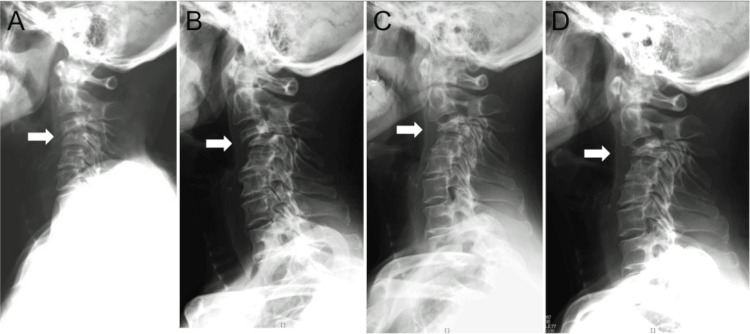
Time Course of Spontaneous Cervical Compression Fracture Progression The spontaneous cervical compression fracture exhibits progression over the span of three years, accompanied by the emergence of kyphotic changes (A: Initial X-ray image, B, C, and D: Images captured 5 months, 2 years, and 3 years after the initial visit, respectively).
